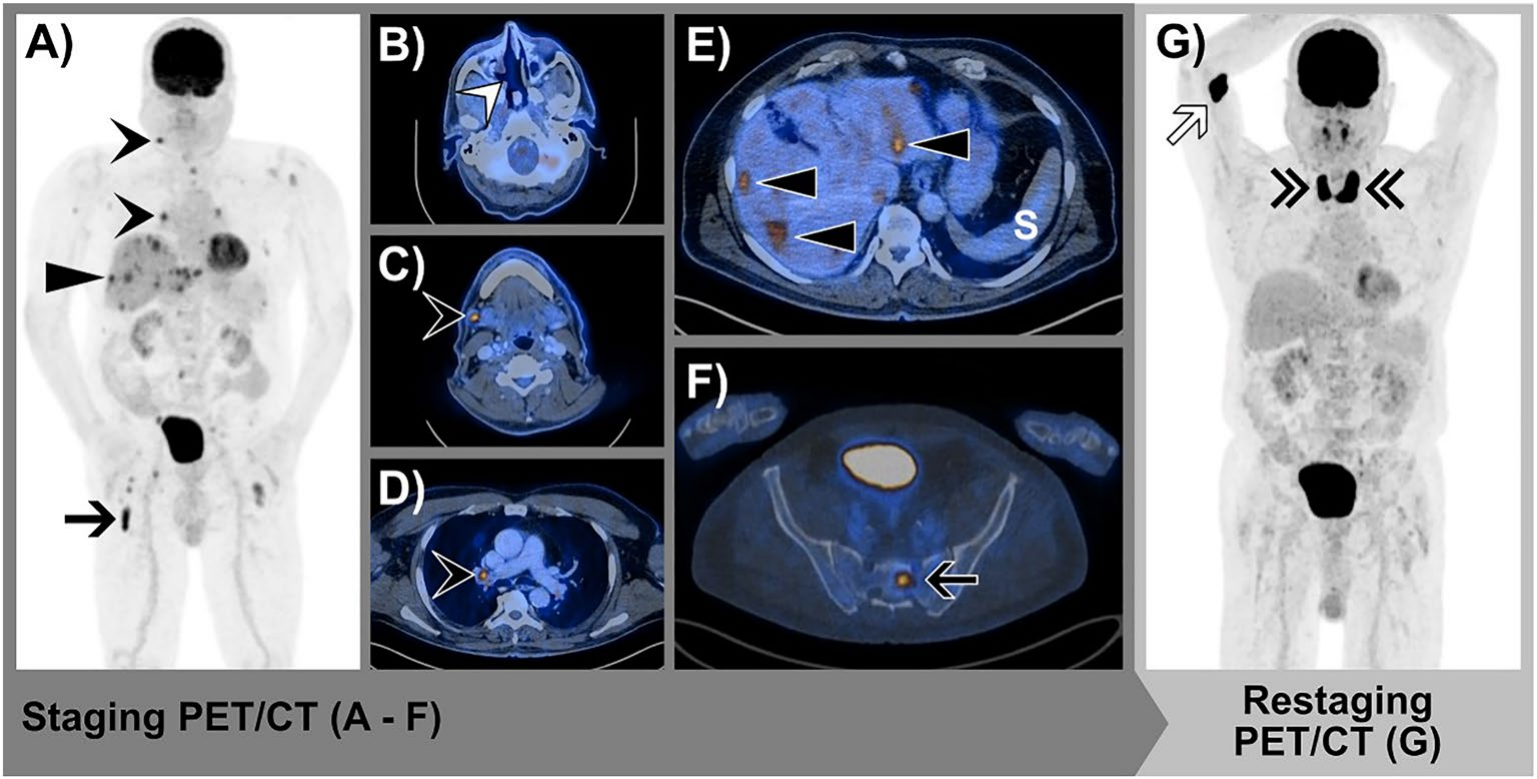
73-year-old male with SMM in the nasal cavity. FDG-PET/CT staging examination before the initiation of anti-PD-1-based therapy and after surgical resection and local RT: (A) Whole-body maximum intensity projection (MIP) image shows multiple cervical and mediastinal lymph node metastases (black arrowheads), multiple liver metastases (black triangle) and multiple bone metastases (black arrow). (B) Axial fused FDG-PET/CT depicted no local tumor persistence in the nasal cavity (white arrowhead). (C) Axial fused FDG-PET/CT with an example of a cervical lymph node metastasis (black arrowhead). (D) Axial fused FDG-PET/CT with an example of a mediastinal lymph node metastasis (black arrowhead). (E) Axial fused FDG-PET/CT with examples of liver metastases (black triangle). (F) Axial fused FDG-PET/CT with an example of a bone metastasis in the sacral bone. FDG-PET/CT restaging examination after the initiation of anti-PD-1-based therapy: (F) Whole-body maximum intensity projection (MIP) image shows CMR of all metastases. The newly appeared strongly increased uptake in the thyroid gland is consistent with immunotherapy-induced thyroiditis (double black arrowheads). FDG-extravasate at the cubital injection site (white arrow). *CMR* complete metabolic response, *FDG-PET/CT* 2-[^18^F]-fluorodeoxy-d-glucose positron emission tomography/computed tomography, *RT* radiation therapy, *S* spleen, *SMM* sinonasal mucosal melanoma.
